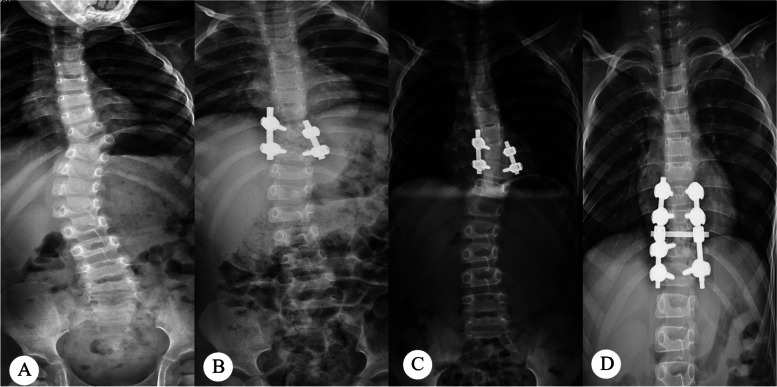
Male child, 5.5 years of age at first operation, diagnosed with T9 hemivertebra. A Preoperative anteroposterior X-ray; B Postoperative anteroposterior X-ray; C Last follow-up anteroposterior X-ray; D Anteroposterior X-ray reexamination after secondary revision surgery
